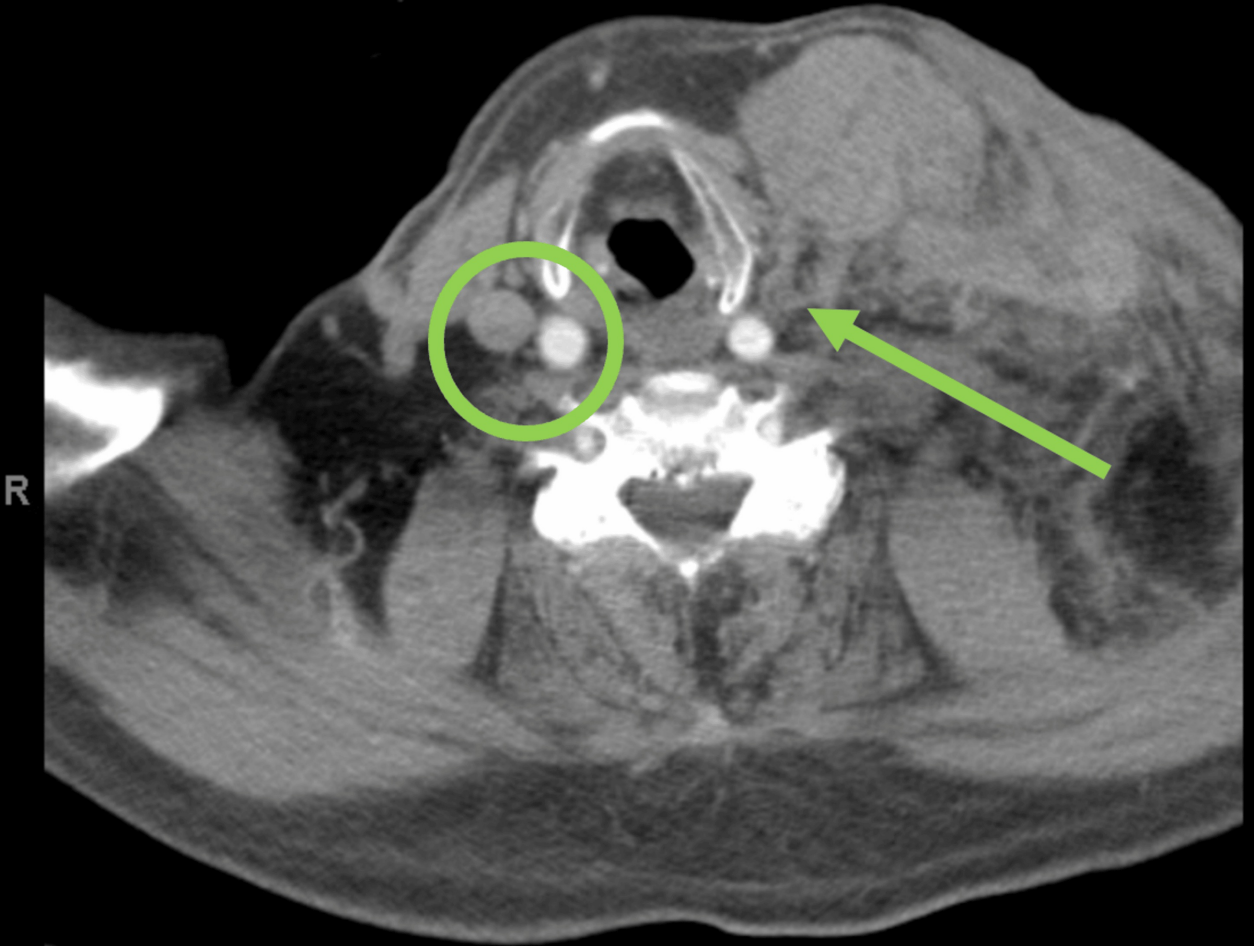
Neck computer tomography angiogram. Neck CTA demonstrating opacified bilateral carotid arteries, patent right internal jugular vein (green circle), and disrupted left internal jugular vein along with massive left neck hematoma (green arrow). CTA: computer tomography angiogram.
